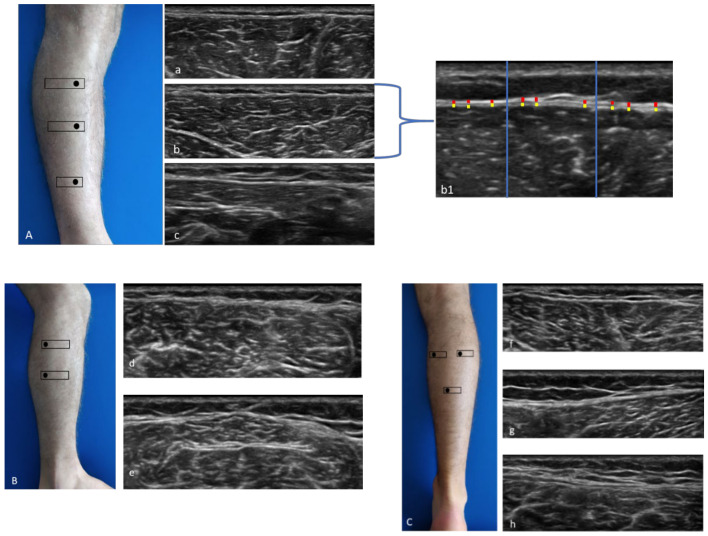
Ultrasound (US) images of the anterior (A), lateral (B), and posterior (C) compartments. Anterior compartment (A) at the levels Ant 1 (a), Ant 2 (b), and Ant 3 (c); method of measurement (b1). Lateral compartment (B) at levels Lat 1 (d) and Lat 2 (e). Posterior compartment (C) at the levels Post 1 (f), Post 2 (g), and Post 3 (h). Probe: black rectangle. Yellow dashes: epimysial fascia; red dashes: crural fascia.
